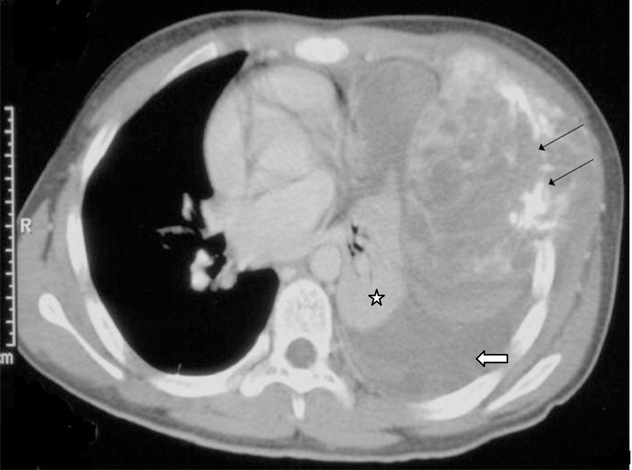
A contrast enhanced CT examination of the chest showing a large heterogenously enhancing solid mass arising from the skeletal chest wall with lytic destruction of the rib and calcifications (arrows). There is a moderate-sized pleural effusion (block arrow) and underlying lung collapse and consolidation (star).
